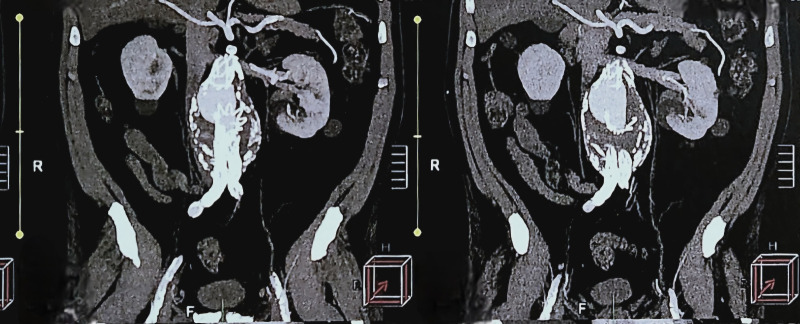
Showing the type IIIa endoleak in coronal computed tomography images, without contrast, in 2016.
